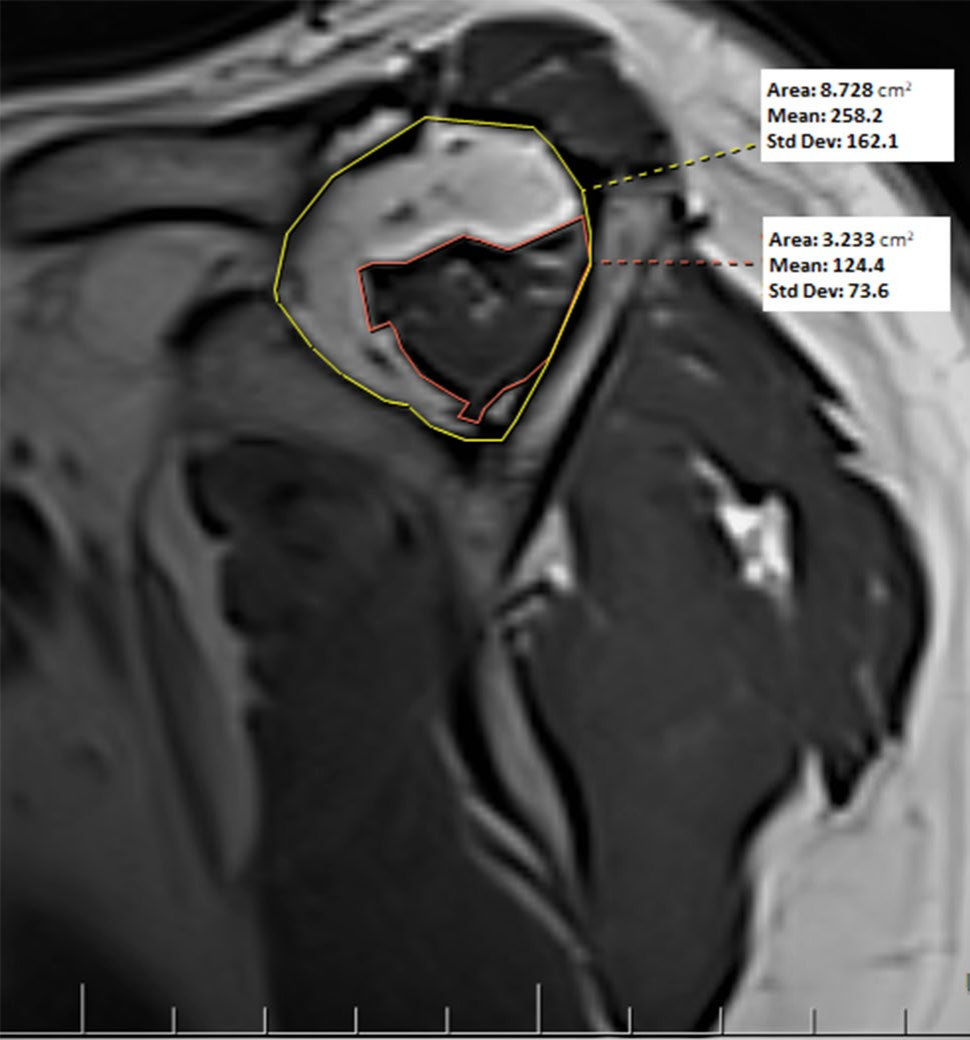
Supraspinatus muscle atrophy measurement on a T1-weighted oblique sagittal MRI slice. In the given patient sample, atrophy was calculated as 63% (8.73–3.23/8.73)
